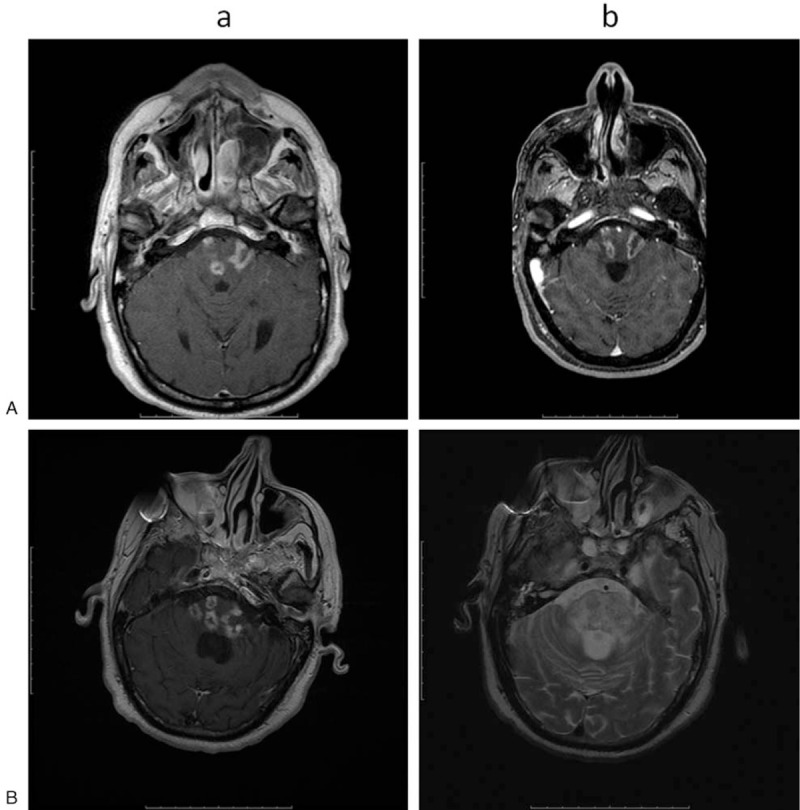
(A) Brain MRI at T10 showing multiple, nodular or curvilinear brainstem white matter lesions characterized by low signal in T1 (a) with heterogeneous contrast enhancement (“Swiss-cheese"), and high signal in T2 (edema and mass effect) (b). (B) Brain MRI at T11 showing more brainstem lesions with a worsening of the MRI features (edema and contrast enhancement). MRI = magnetic resonance imaging.
